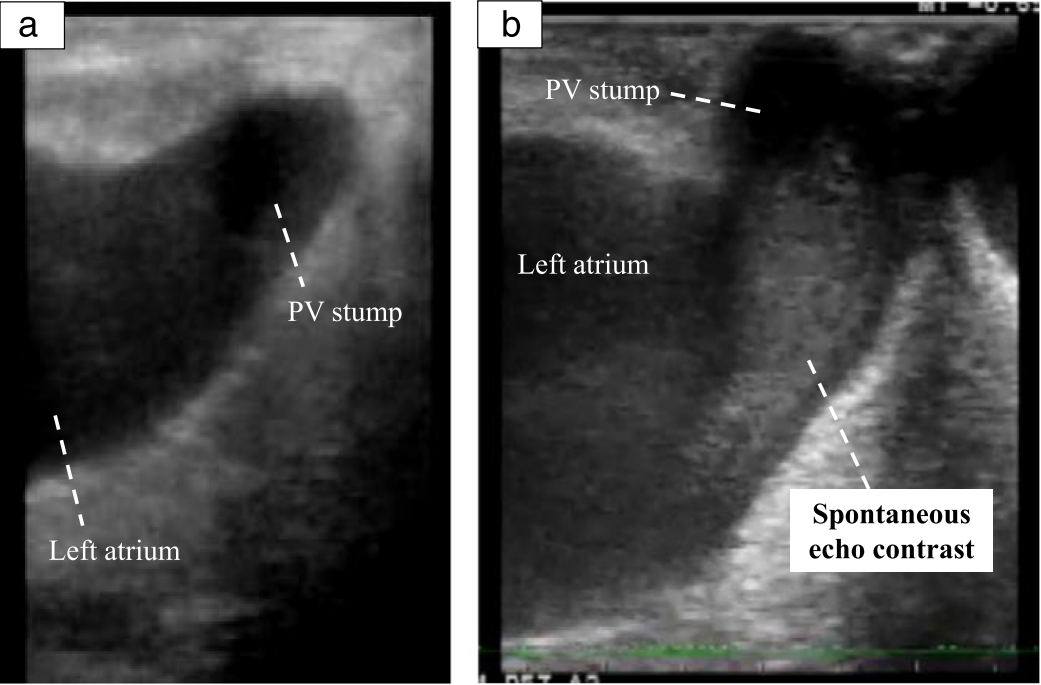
Spontaneous echo contrast in the pulmonary vein stump. Ultrasonographic images in the left superior pulmonary vein stump show the absence (a) and the presence of spontaneous echo contrast (b). PV: Pulmonary vein.
